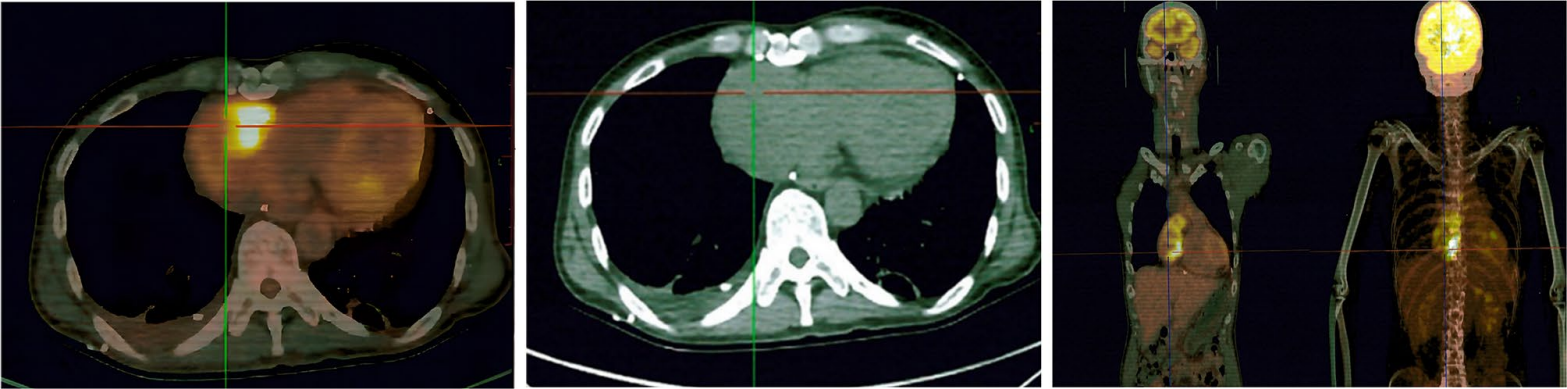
Preoperative 18-fluorodeoxyglucose positron emission tomography-computed tomography(18FDG-PET/CT). A mass was observed in the right atrial area of size approximately 6.5 * 5.9 cm.The maximum standardized uptake value (SUVmax) was 14.2
